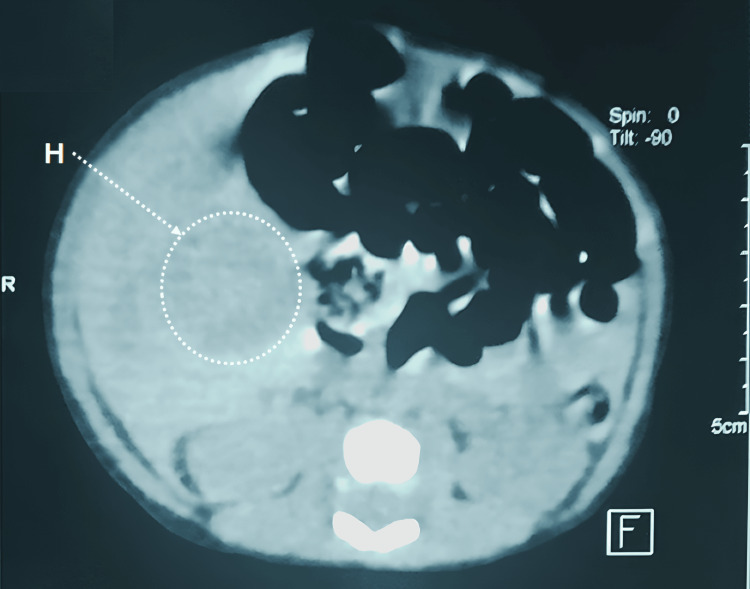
Abdominal-pelvic CT scan showing a hepatic hematoma (H: Borders of the hematoma)
